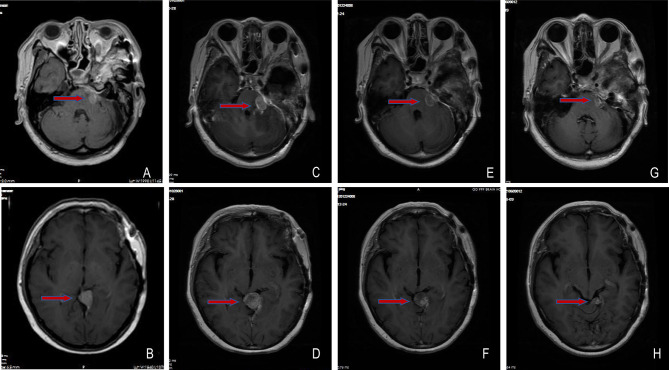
Changes of meningioma at different shooting angles of MRI. (A, B) Meningioma recurred on 2020-07-23; (C, D) The lesions in the left cerebellopontine angle area enlarged on 2020-10-28; (E, F) The residual lesions lessened on 2020-12-24; (G, H) The residual lesions continuously reduced on 2021-06-09. The red arrow points to the tumor.
